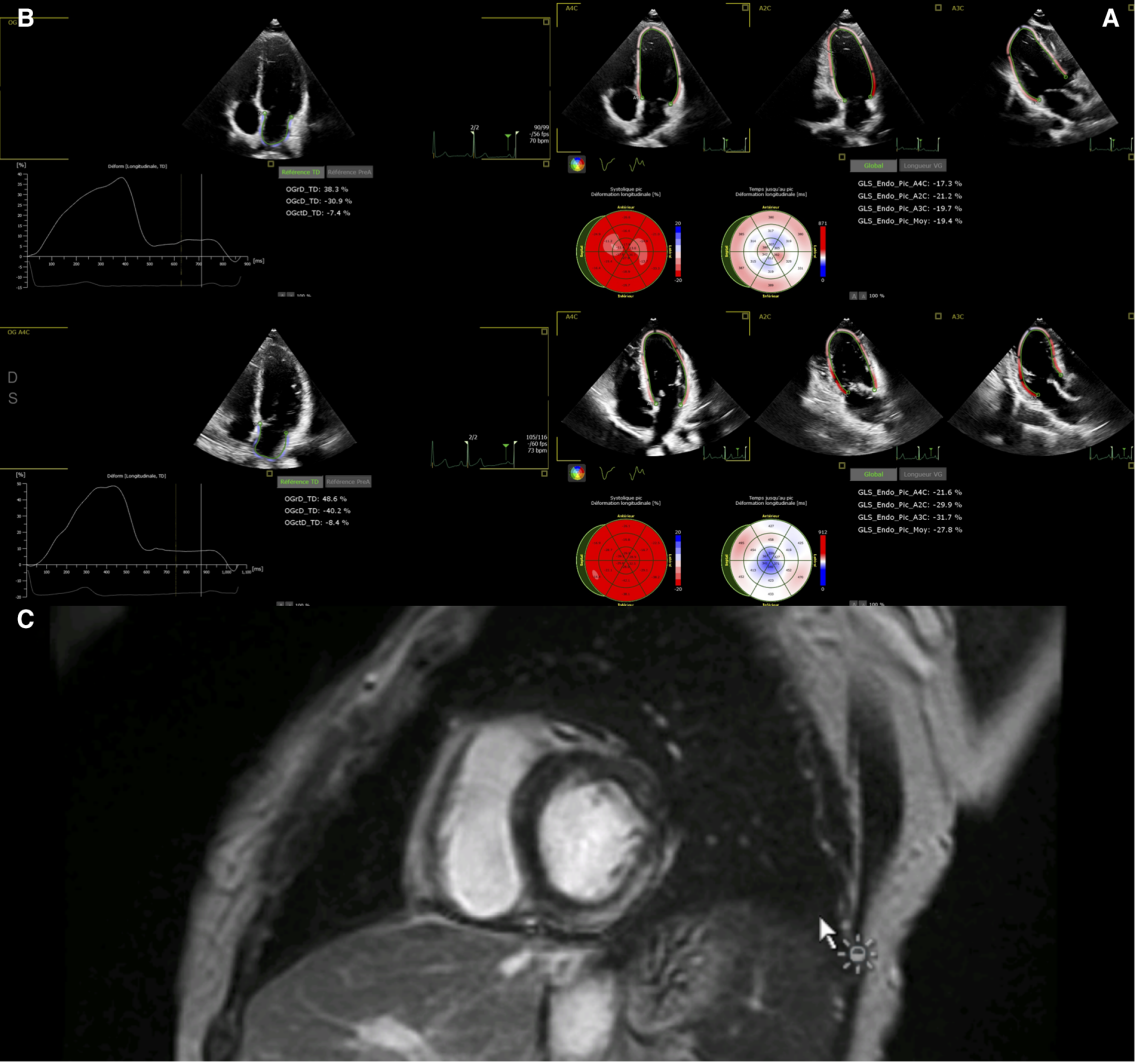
(A) Global left ventricular longitudinal strain in a 12-year-old boy before discharge (upper panel) and after 6 months. (B) Left atrial strain in the same 12-year-old boy before discharge (upper panel) and after 6 months. (C) Cardiac MRI in a 7-year-old boy 3 days after admission, showing late gadolinium enhancement within the inferior and lateral wall.
